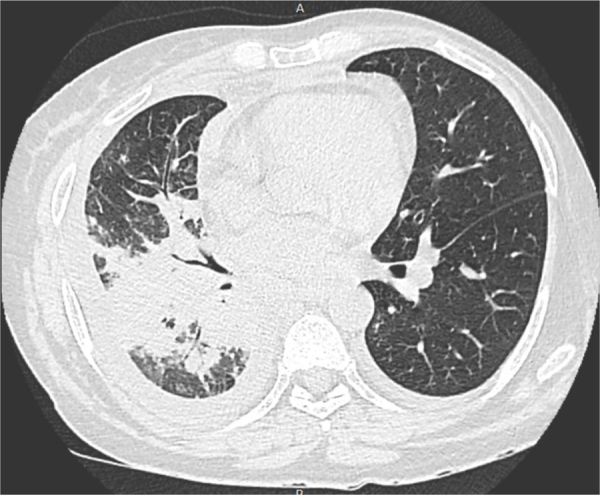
Paracoccidioidomycosis and liver transplantation: CT scan showing peribronchial and perivascular parenchymal consolidations.
